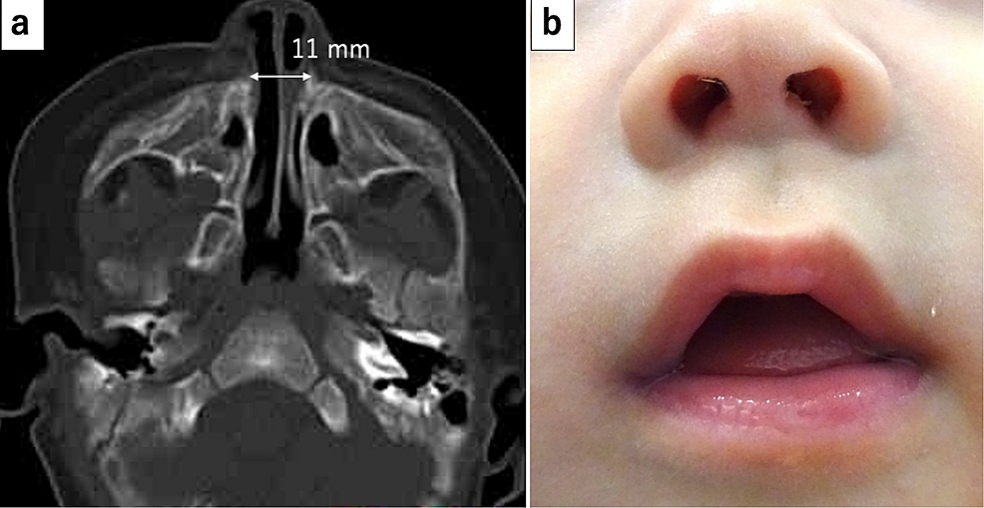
Postoperative findings. (a) Postoperative axial CT scan on the 22nd day postoperatively. The width of the pyriform aperture was 11 mm. (b) Appearance of the nostrils one month after removal of the stents.
